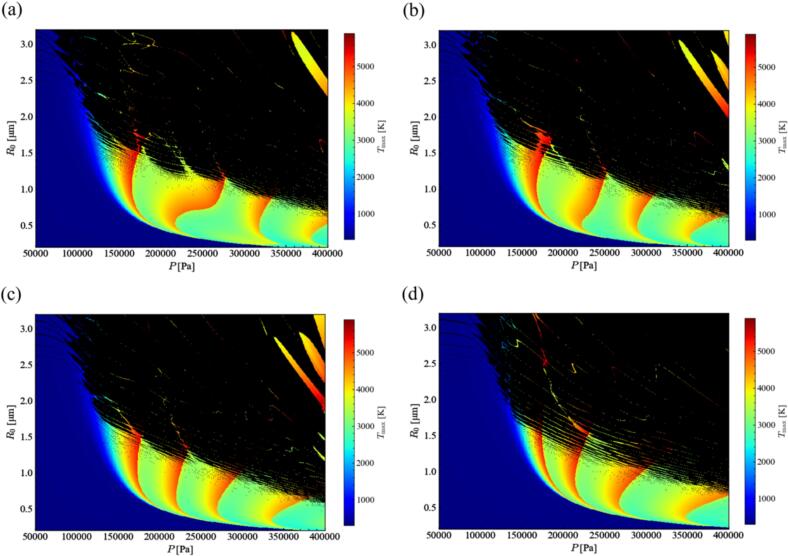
Distribution of the maximum temperature in the bubble in an ultrasonic cycle with different ratio of fundamental frequency, *α*_1_: (a) 0.7, (b) 0.8, (c) 0.9, and (d) 1.0. The black zone indicates the unstable zone, where the stable bubble cannot exist.
